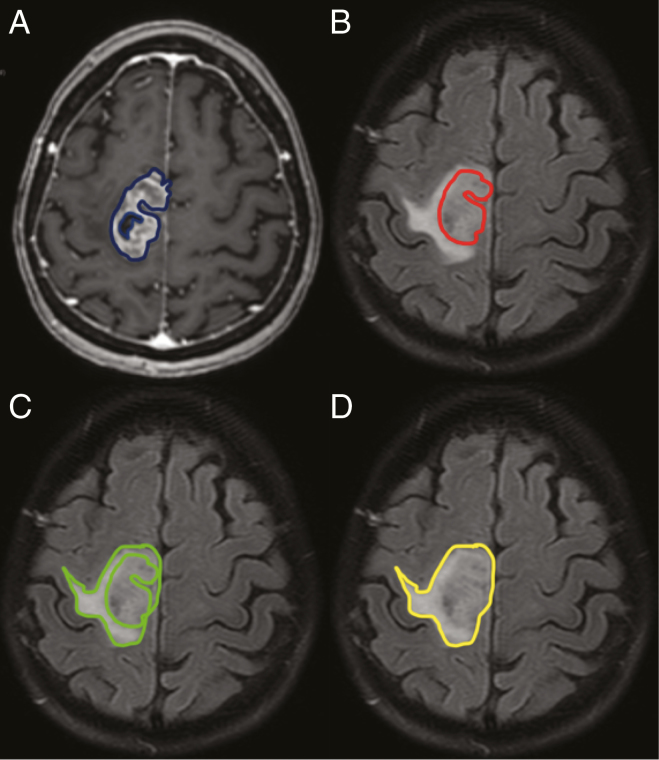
Example of MRI segmentation. Segmentation of the contrast-enhanced tumoral portion using T1-weighted contrast-enhanced images (A–blue color) and fluid-attenuated-inversion-recovery (FLAIR) images (B–red color); of the peri-tumoral parenchymal area using FLAIR (C–red color); and of a volume including the contrast-enhanced tumoral portion and the peri-tumoral parenchymal area (D–yellow color).
